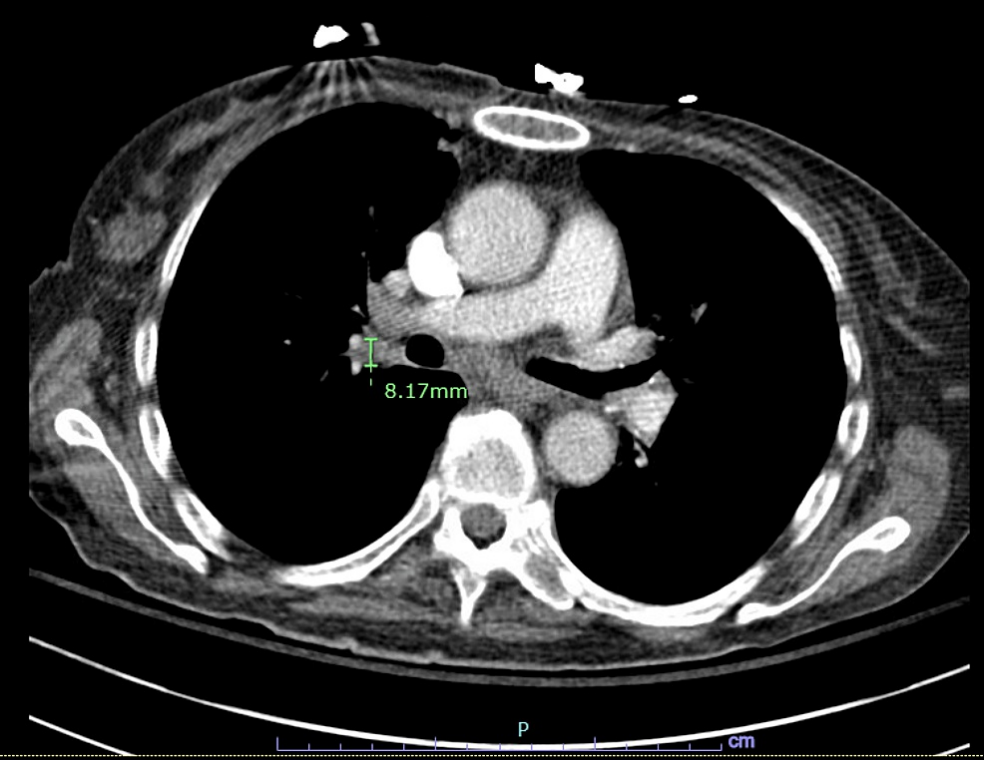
Computed tomography (CT) scan of the chest with contrast showing hilar adenopathy (green measurement)
